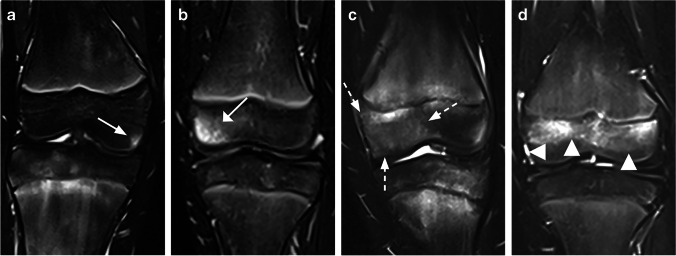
Coronal water-only Dixon T2-weighted magnetic resonance images in (a) a 14-year-old girl with an area of increased signal intensity in the epiphysis of the femur with an extension up to 5% (*arrow*), (b) a 7-year-old girl with an area of increased signal intensity in the epiphysis of the femur with an extension up to 1/3 (*arrow*), (c) a 14-year-old boy with an area of increased signal intensity in the epiphysis of the femur with an extension up to 2/3 (*dotted arrows*) and (d) a 7-year-old girl with an area of increased signal intensity in the epiphysis of the femur with an extension up to 3/3 (*arrowheads*)
